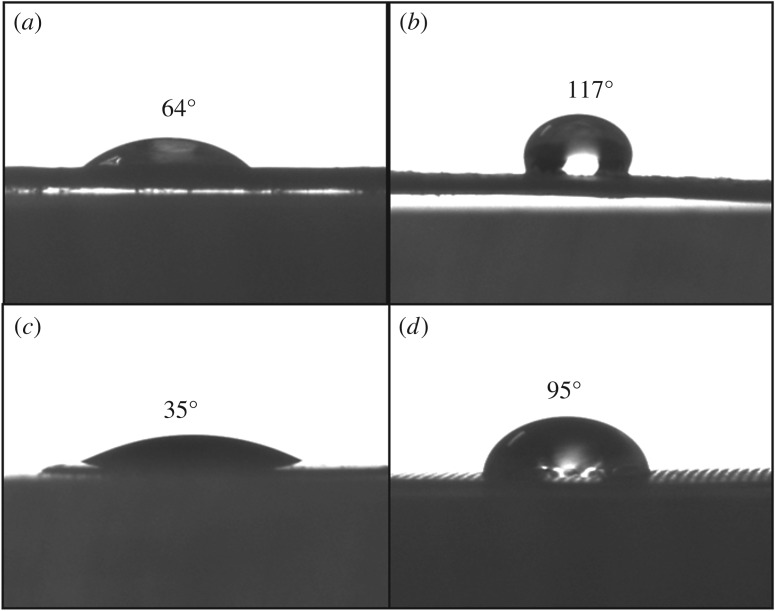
Contact angle: (*a*) active layer of CTA-NW; (*b*) support layer of CTA-NW; (*c*) active layer of TFC-ES and (*d*) support layer of TFC-ES.
